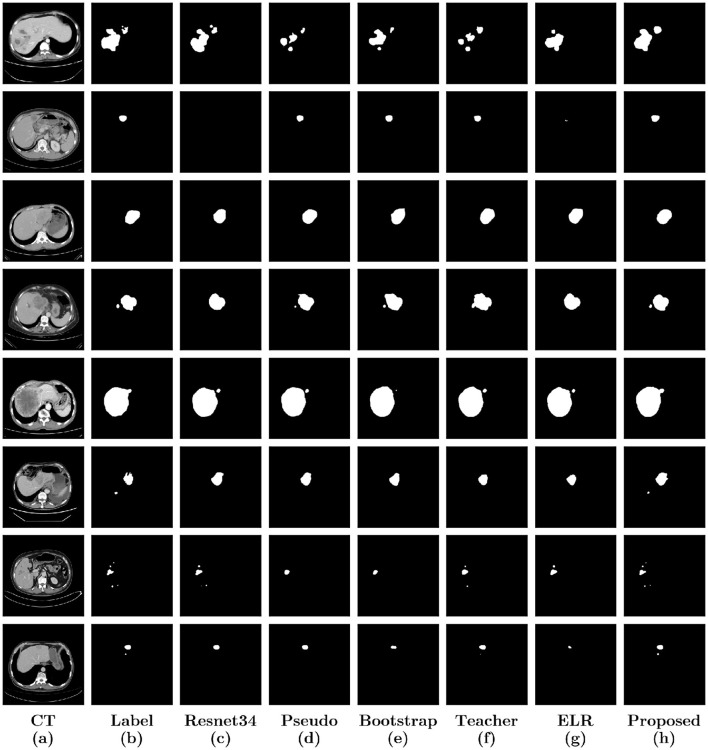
Display of results of liver tumor segmentation by different methods. Eight samples were selected to display the results of the six methods. (a) Original CT images; (b) Labels; (c) Results of U-Net with a backbone structure Resnet34; (d) Results of training with pseudo label; (e) Results of hard bootstrapping; (f) Results of training with mean teacher; (g) Results of training with ELR loss; (h) Results of our method.
